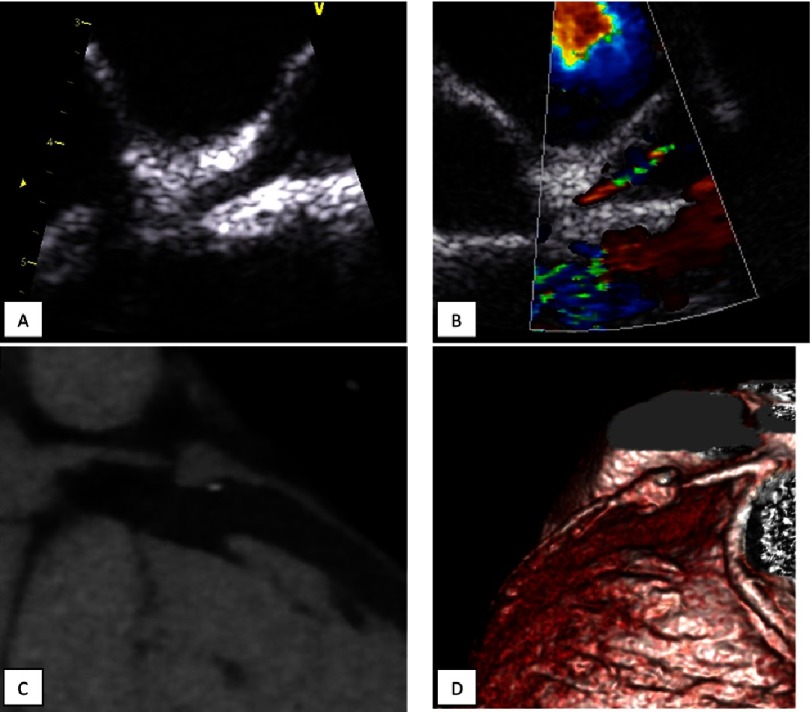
A subtotal stenosis at the entrance of an aneurysm of the LAD 2,67 years after the onset of KS in a boy who suffered KS at the age of 1,58 years. A – echocardiogram. A significant thickening of aneurysmal walls in LAD especially at its entrance. B – echocardiogram. Aliasing phenomenon can be seen at the entrance of an aneurysm in color flow mode that reflects a high-speed flow. The flow rate in LAD before the aneurysm in PW mode was 0,4 m/s while at the entrance of an aneurysm it exceeded 1,5 m/s. C, D - MDCT, C - multiplanar reformation, D - three-dimensional reconstruction. A subtotal stenosis at the entrance of an aneurysm. Calcification can be seen in the walls of an aneurysm.
